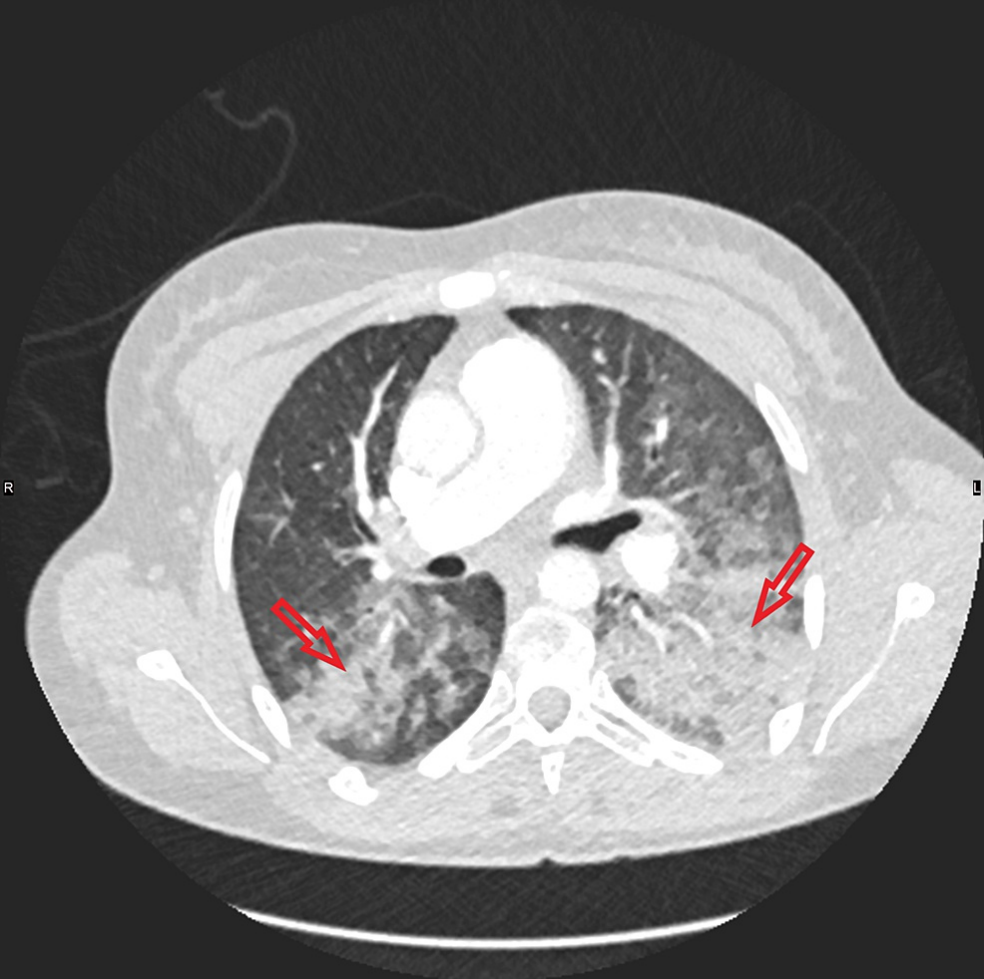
Computed tomography scan showing bilateral ground-glass opacities.
